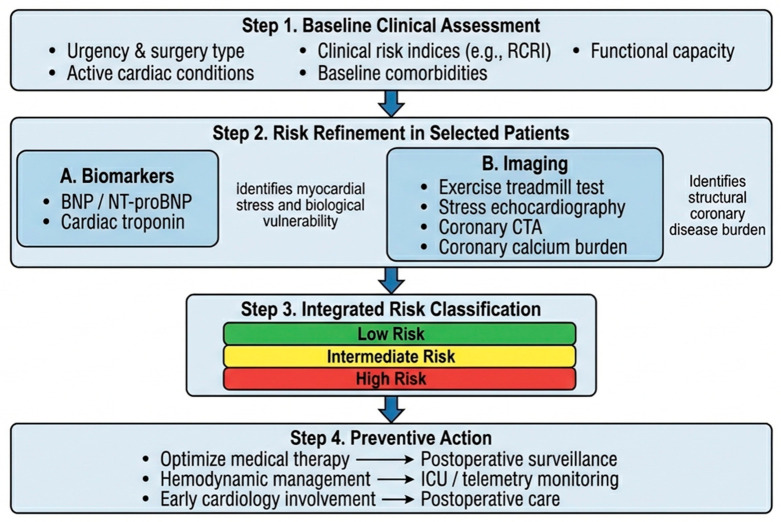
Proposed integrated framework for perioperative myocardial infarction risk stratification and prevention in patients undergoing noncardiac surgery. Perioperative cardiovascular evaluation begins with conventional clinical assessment, including surgical risk, active cardiac conditions, comorbidities, and functional capacity. In selected patients, biomarkers such as natriuretic peptides help assess myocardial vulnerability, whereas imaging modalities, particularly coronary computed tomography angiography, define the structural burden of coronary artery disease. Integrating clinical, biomarker, and imaging data may improve identification of high-risk patients and support individualized preventive strategies, including optimization of medical therapy, hemodynamic stabilization, postoperative troponin surveillance, and intensified perioperative monitoring, while emphasizing that multimodal testing should be selective and should not delay urgent surgery.
